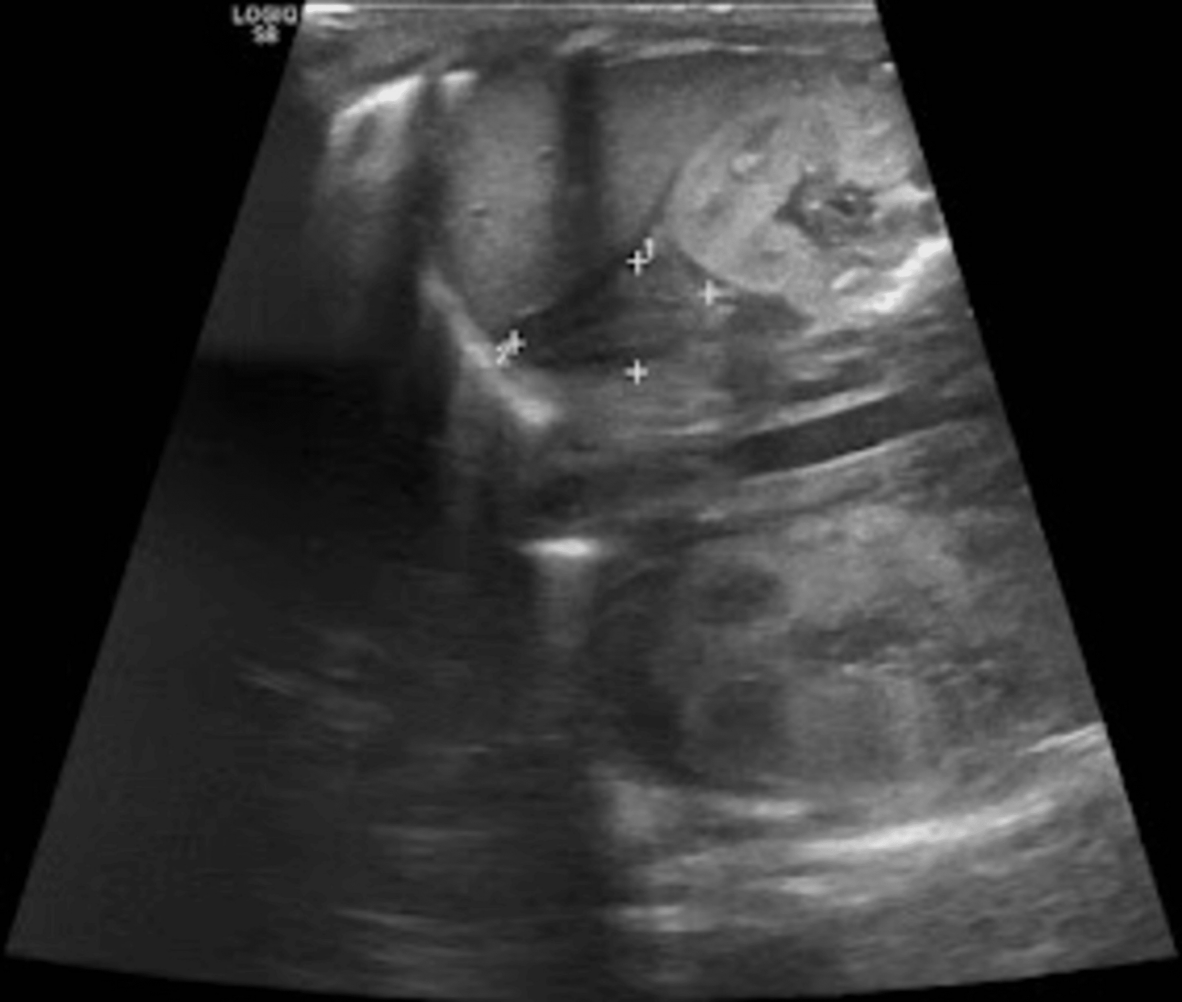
Abdominal ultrasound (10 minutes later) showing the size of right suprarenal gland decreased to 1.81 x 0.99 cm.
